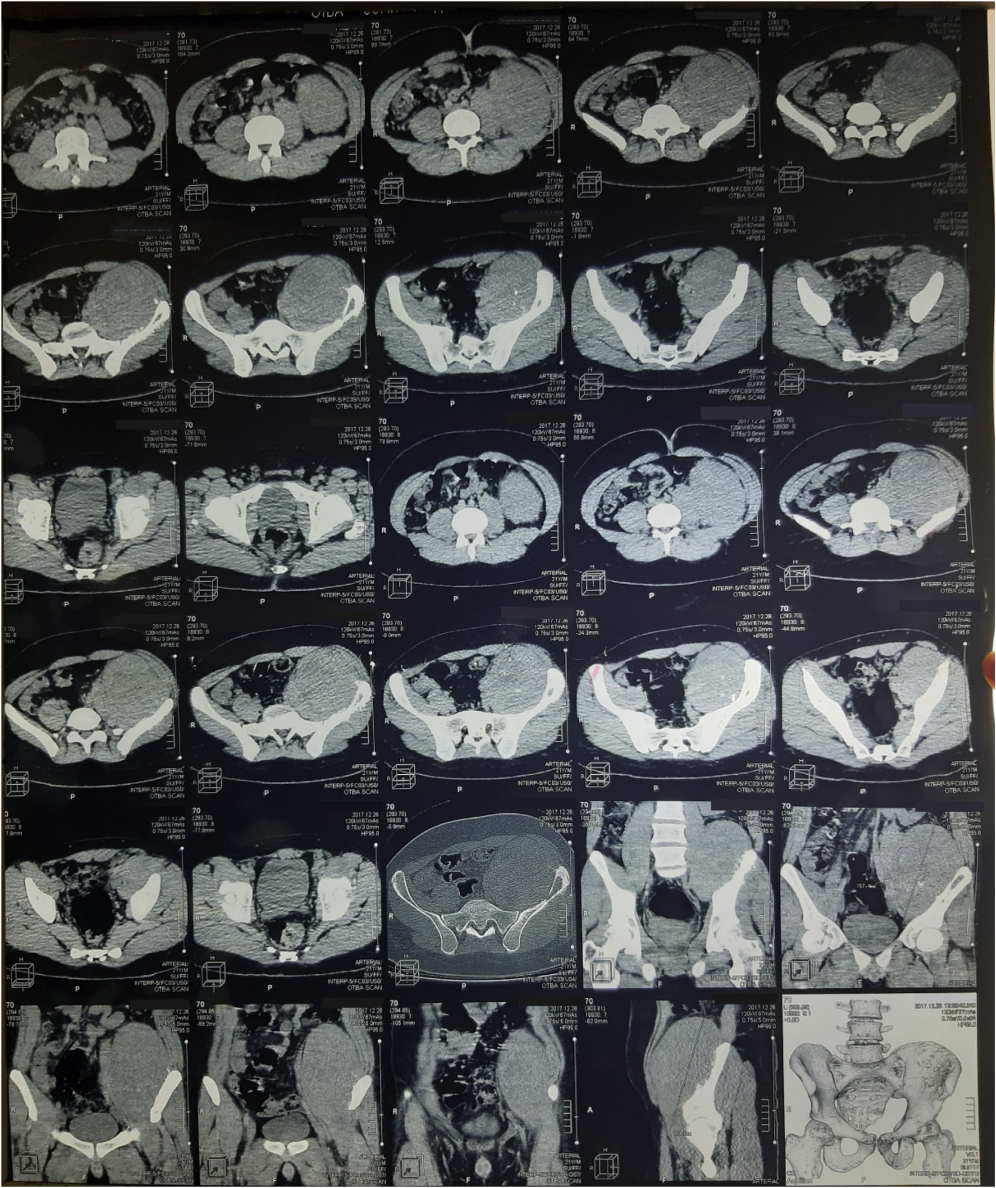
A CT scan showing a huge mass in the left iliac fossa.
